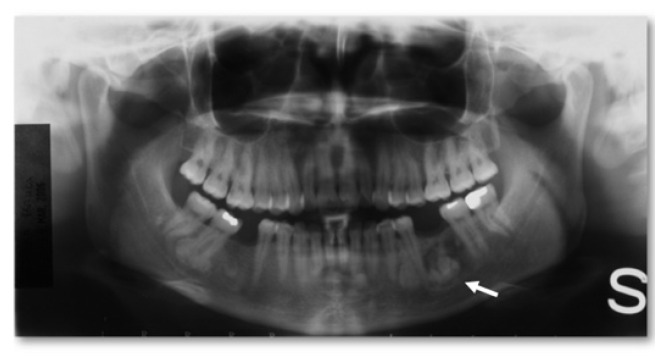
Central osteoma: panoramic radiograph showing a localized, well-defined radiopacity involving the alveolar bone of the left mandibular body (white arrow). The finding was incidental, and the patient did not refer to any symptoms.
